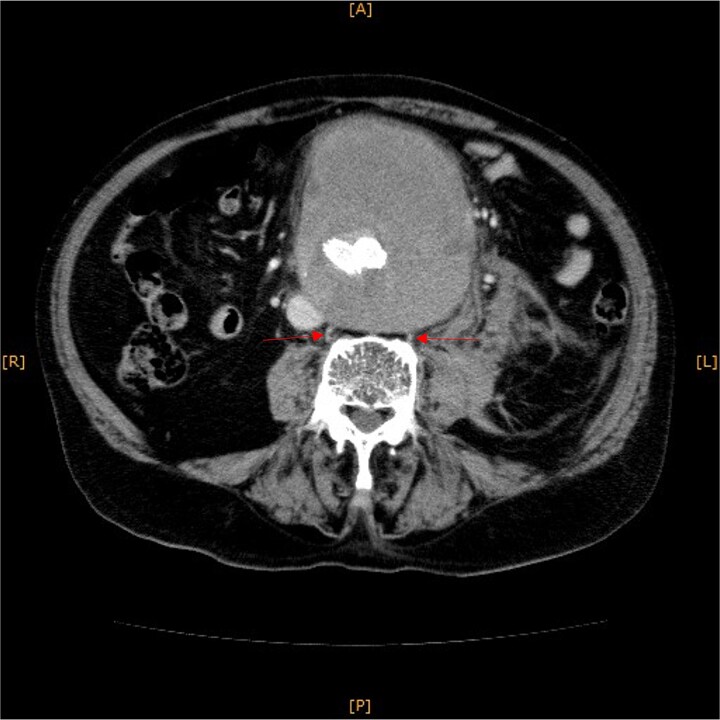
Contrast-enhanced CT revealed an AAA measuring 118 × 107 mm and left retroperitoneal hematoma. One pair of lumbar arteries was enhanced only in the delayed phase (red arrows). Intraoperatively, pulsatile bleeding was observed from the left one of this pair.
